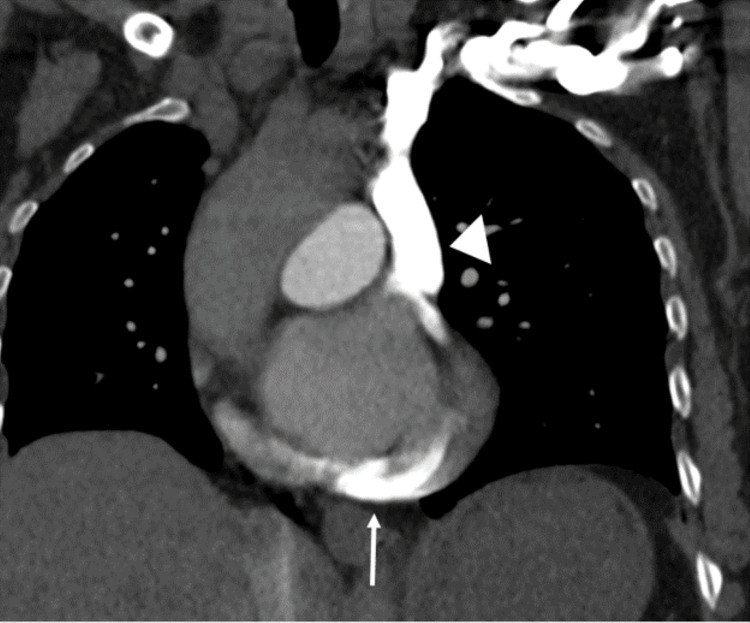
Coronal MIP image of the chest from the second CT angiogram injected through the left upper extremity The contrast bolus is seen entering the right atrium via the left-sided SVC (white arrowhead) through the coronary sinus (white arrow). MIP: maximum intensity projection; SVC: superior vena cava.
